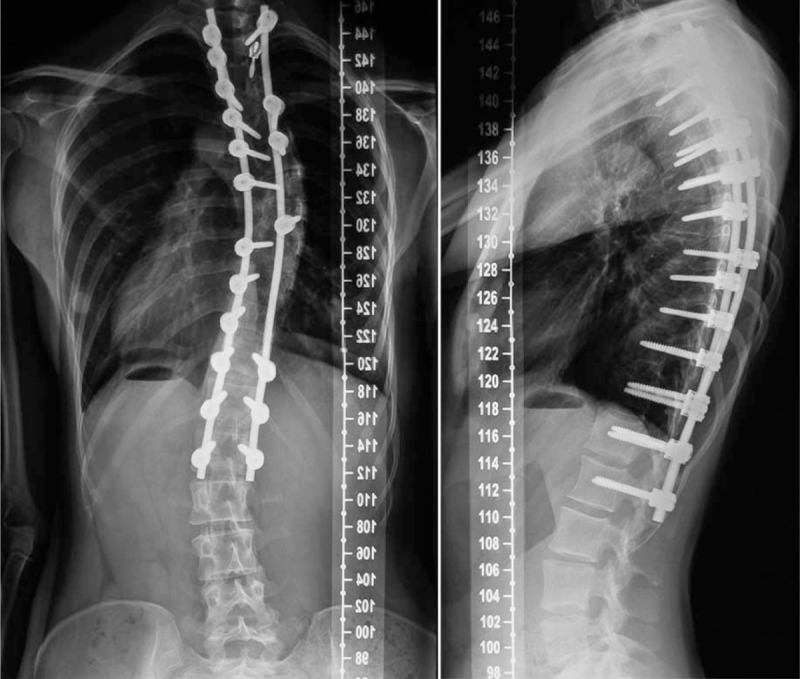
Standing anteroposterior and lateral radiographs of 12 months after operation.
